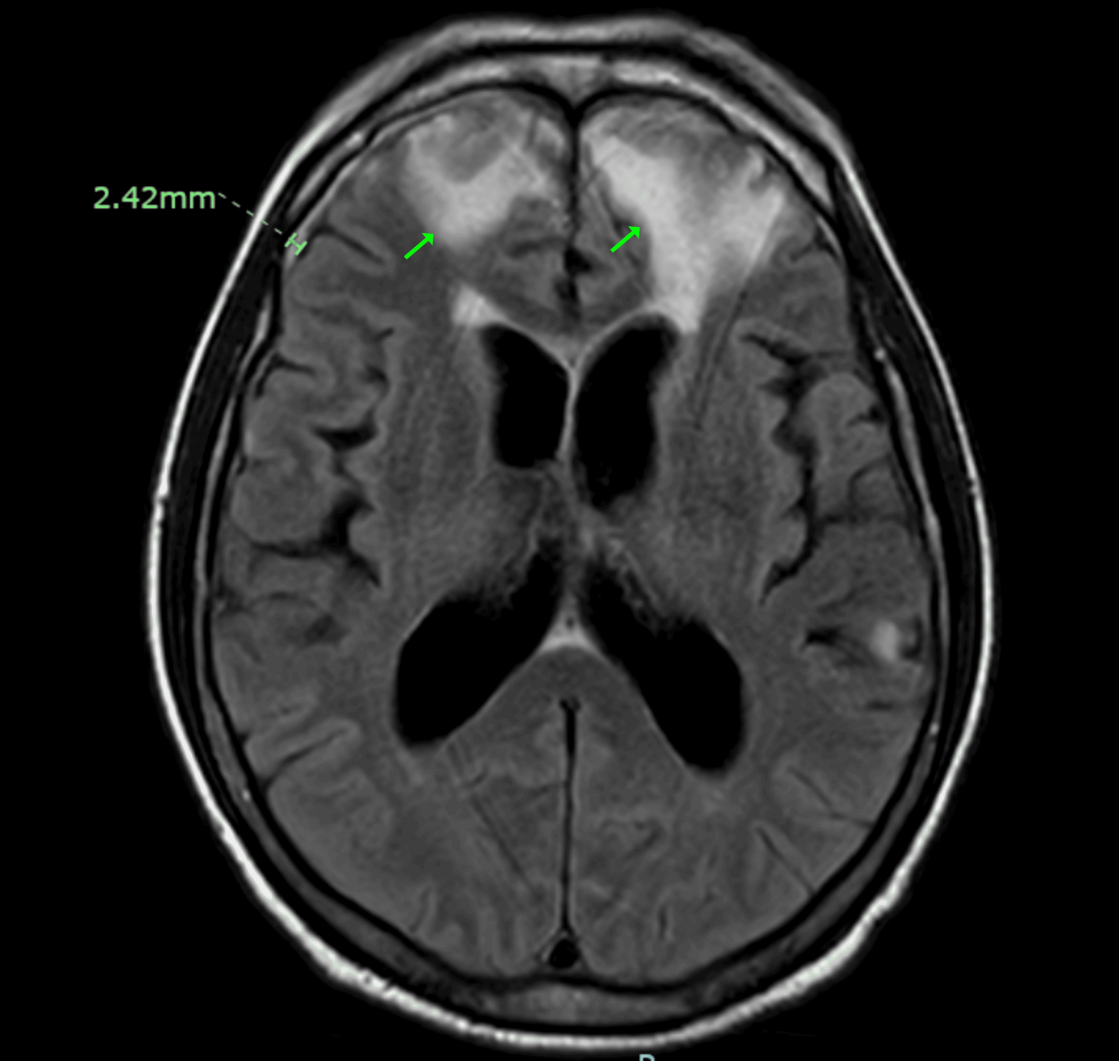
MRI brain with bilateral frontal lobe hyperintensities and right subdural hemorrhage. MRI T2 Fluid Attenuation Inversion Recovery (FLAIR) hyperintensities in bilateral frontal lobes (arrows) as well as a 2.42 mm right convexity subdural hemorrhage (bracket).
